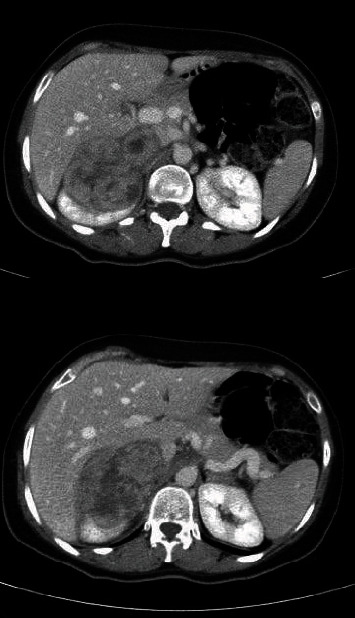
Axial CT views of the lesion, showing the involvement of the right kidney and IVC.
